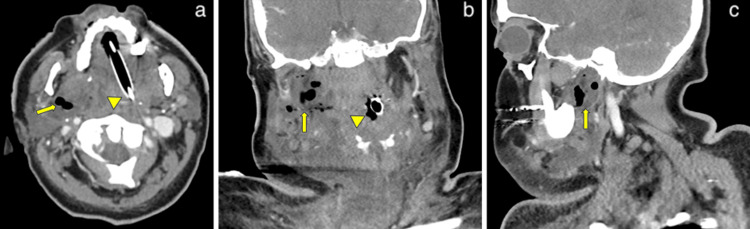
Contrast-enhanced computed tomography demonstrates submandibular edema and right parapharyngeal fluid and gas collection (arrows) at the C1-C2 levels in the transverse (a), frontal (b), and sagittal (c) planes, causing leftward deviation of the trachea (arrow heads).
